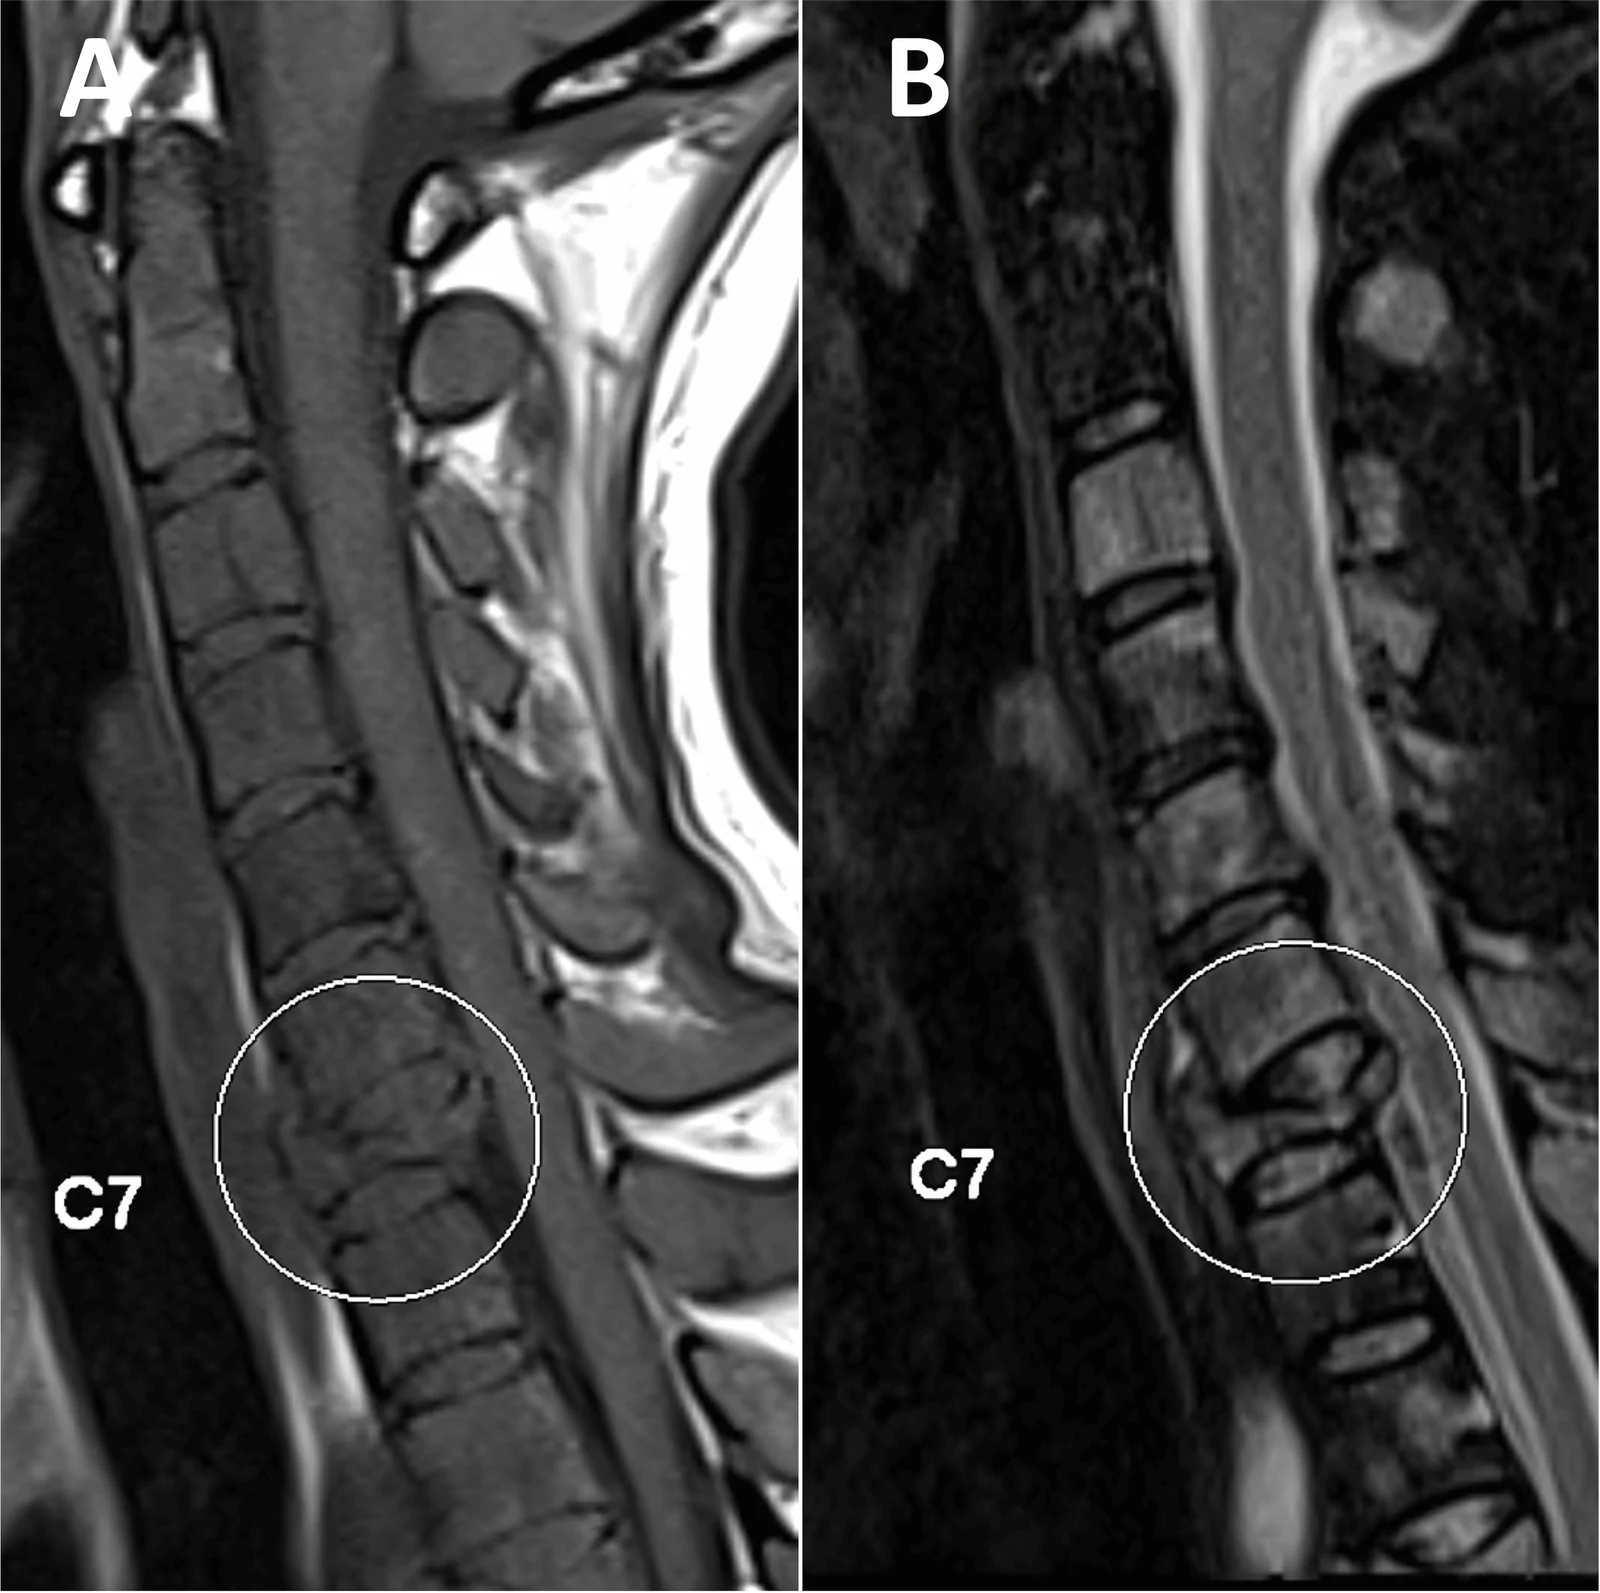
MRI of the cervical spine. (A) T2-weighted image shows the collapse of the C7 vertebra with moderate retropulsion. Moderate stenosis of the central canal is noted. (B) T1-weighted shows abnormal marrow signal involving C2 to C7. Findings are suspicious for a marrow infiltrative process; in particular, bone metastasis merits consideration.
